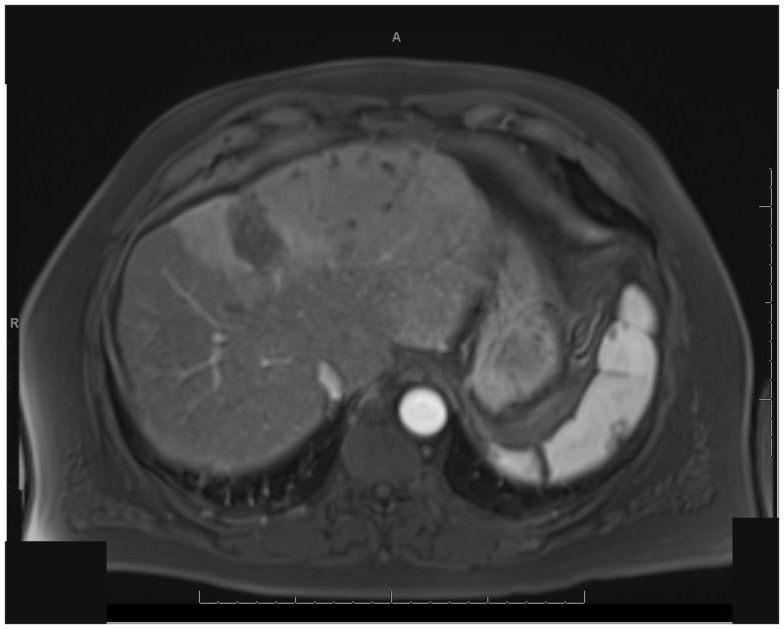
T1 MRI with early phase contrast. Fifteen weeks post-RE.
